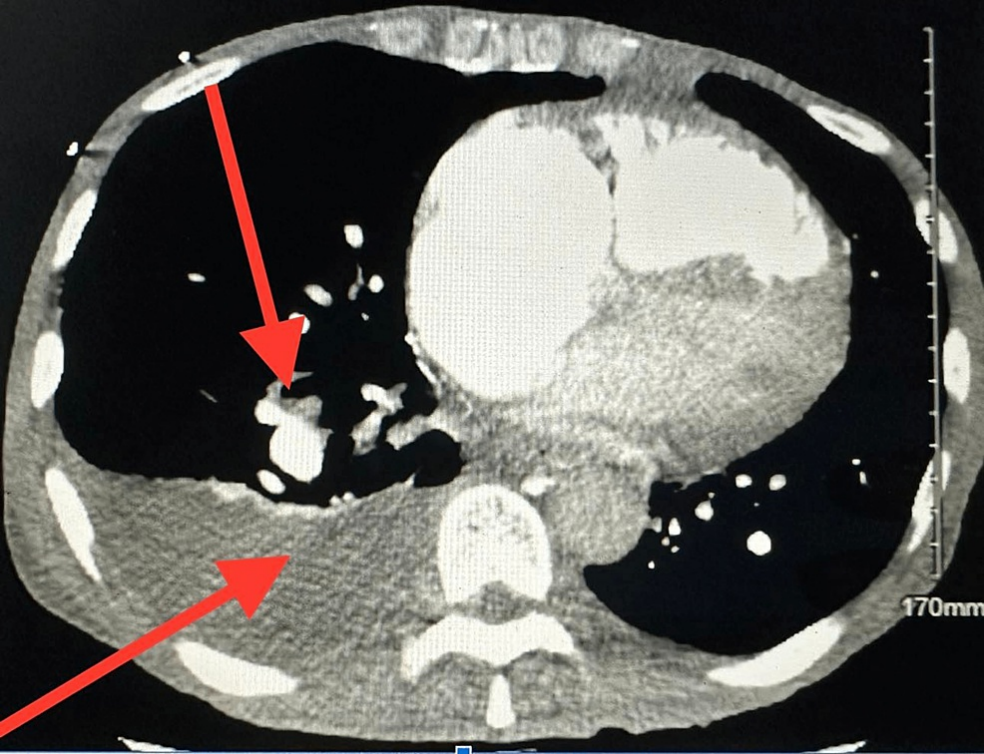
Repeat CTA during readmission showing chronic pulmonary embolus unchanged of descending right interlobar pulmonary artery (red arrows) Small right-sided pleural effusion. CTA: computed tomography angiography.
